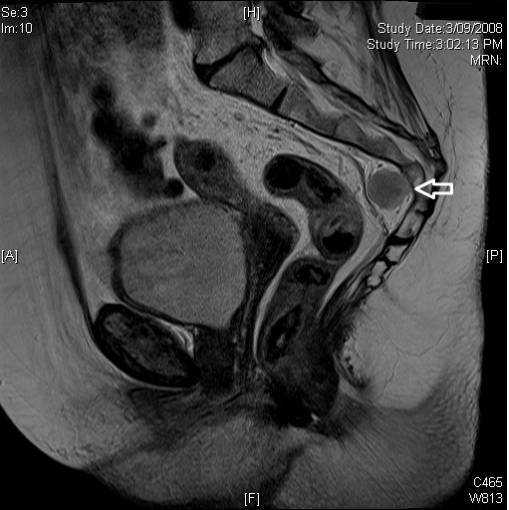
MRI Sagittal view of lesion.
